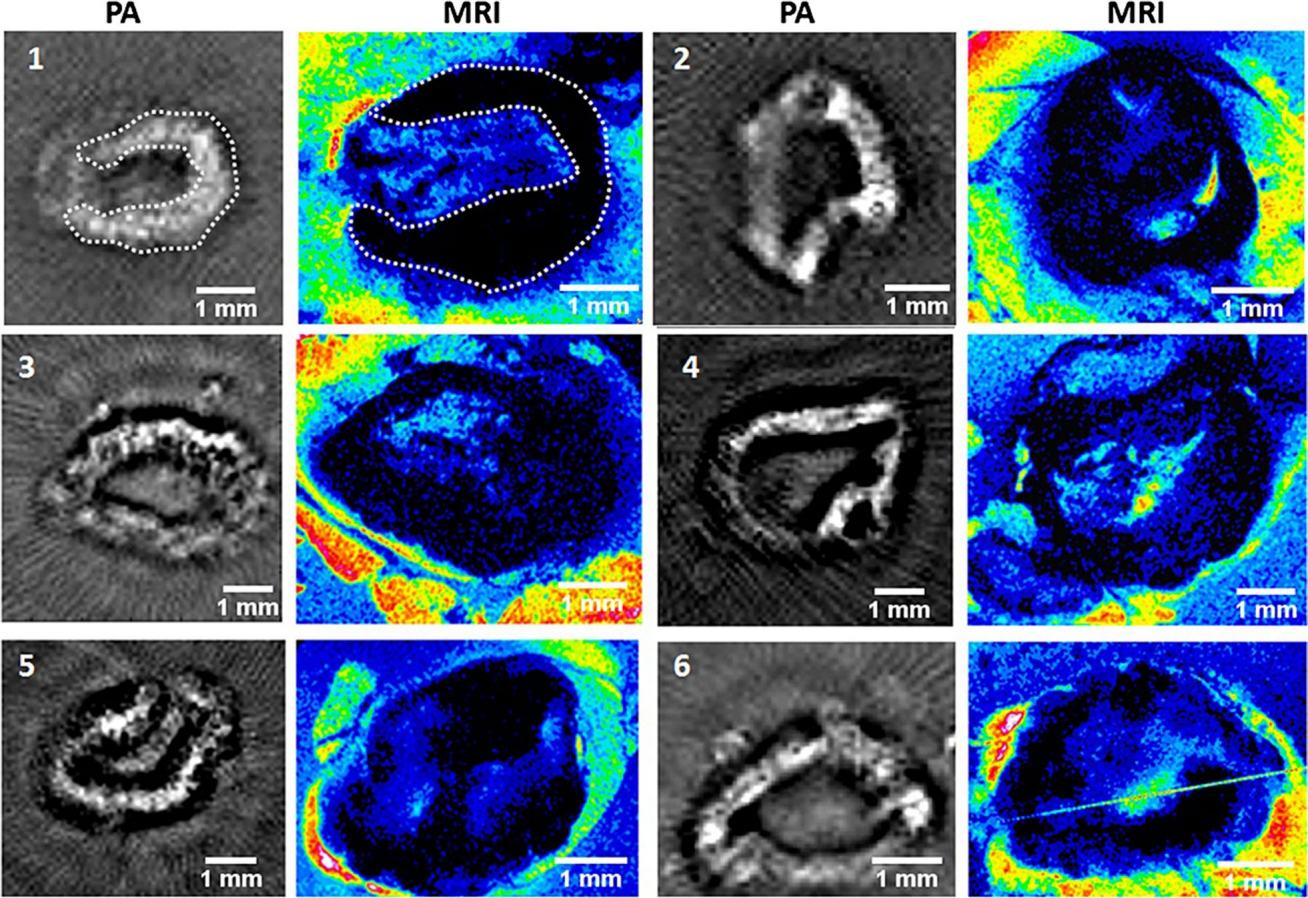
Photoacoustic and MR image comparison of all resected lymph nodes with contrast injection. As shown in lymph node 1 (white dotted line), the PA response pattern is comparable with the location of MRI signal decrease. Some nodes show a continuous contrast band throughout their periphery (1,5), while others show some small irregularities (4,6). The figure was reused from the research article published in the journal Contrast Media and Molecular Imaging by Grootendorst and co-workers in 2013 [190]
